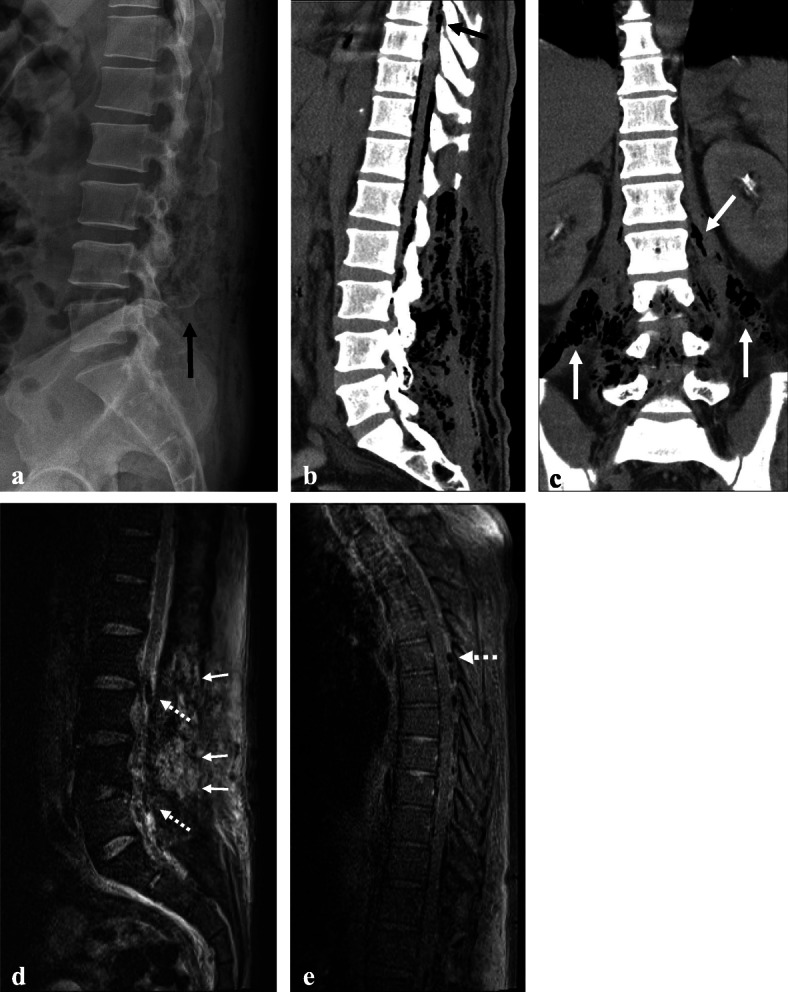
Lateral radiographs (a) showing an aggressive gas-forming lesion around the lumbar paravertebral area (black arrow). Sagittal and coronal computed tomography (b and c) scans showing aggravating subcutaneous emphysema in the psoas muscle (white arrows) and paravertebral muscle as well as in the lumbar epidural space. Sagittal magnetic resonance imaging (d) showing aggressive dissemination of multifocal fluid pockets containing innumerable air bubbles in the paraspinal area, whole lumbar area (white arrows), and epidural space (white dotted arrows), which disseminated into the T5 level (white arrow) (e)
